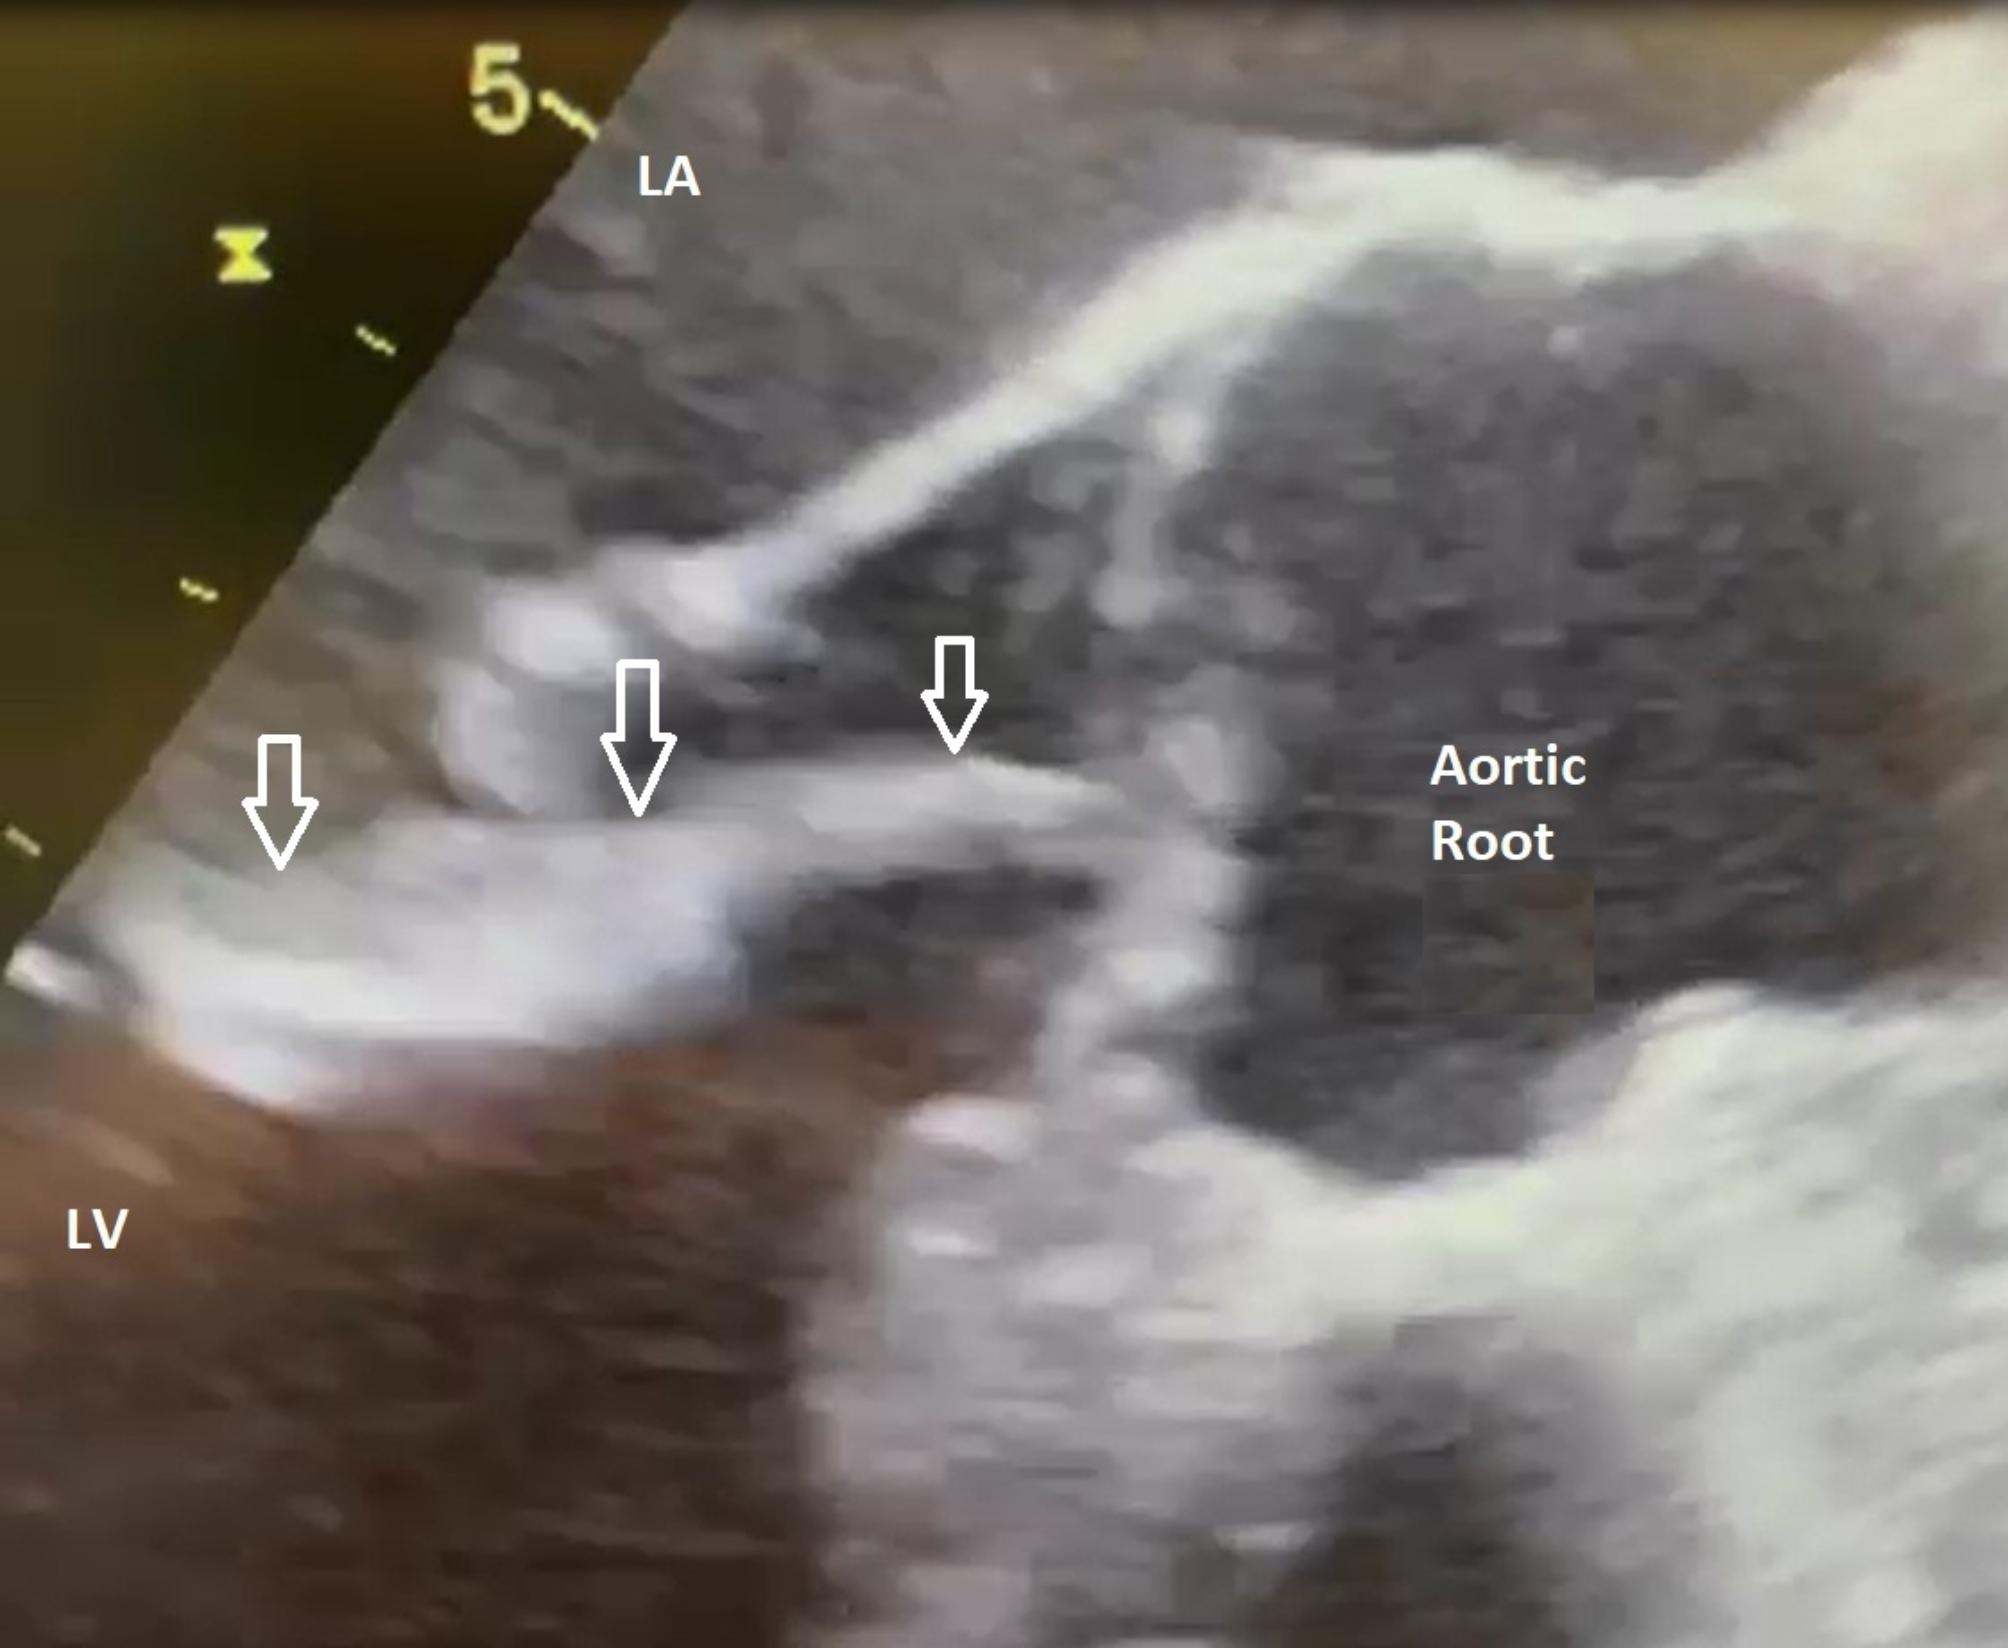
Two dimensional transoesophageal echocardiography (TEE), midesophageal aortic valve long axis view, revealed fluctuating mobile pedicle vegetation attached to the free edge of the right coronary leaflet of the aortic valve
